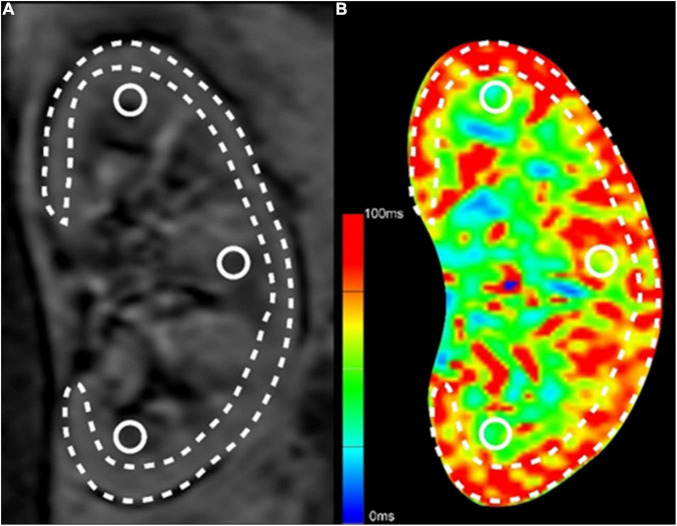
Methods for ROI selection on BOLD-MRI images. (A) The original T2*-weighted image; (B) The pseudo-color T2*-weighted image. The long and narrow area depicted by the dashed line was the ROI of renal cortex, excluding the renal medulla, collecting system, incidental cysts, and hilar vessels. The three circular areas on the upper, middle and lower medulla of the kidney were the ROIs of the medulla.
